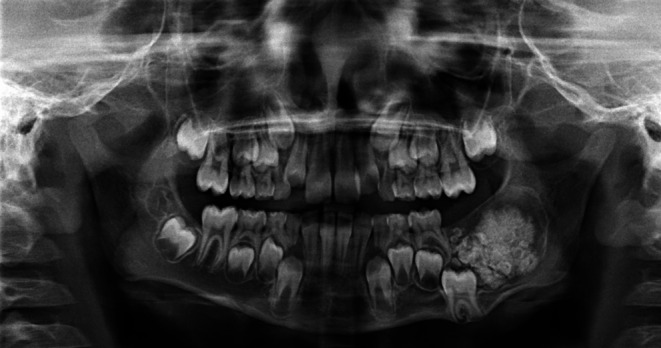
A panoramic radiograph obtained during the initial visit showed a circumscribed unilocular lesion in the lower left mandible containing partly amorphous and partly tooth-like radioopacities. Note the caudally displaced first permanent lower left molar and an absence of the tooth bud of the second lower left molar
